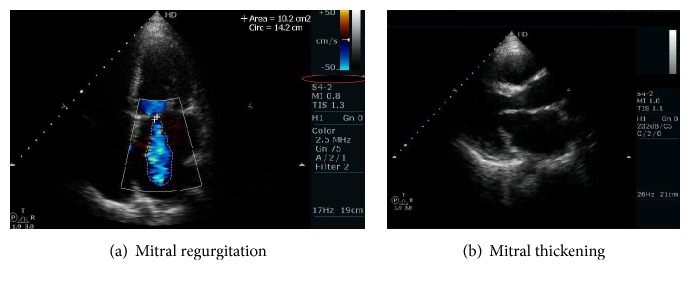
Echocardiography of mitral regurgitation (a) and mitral thickening (b).
